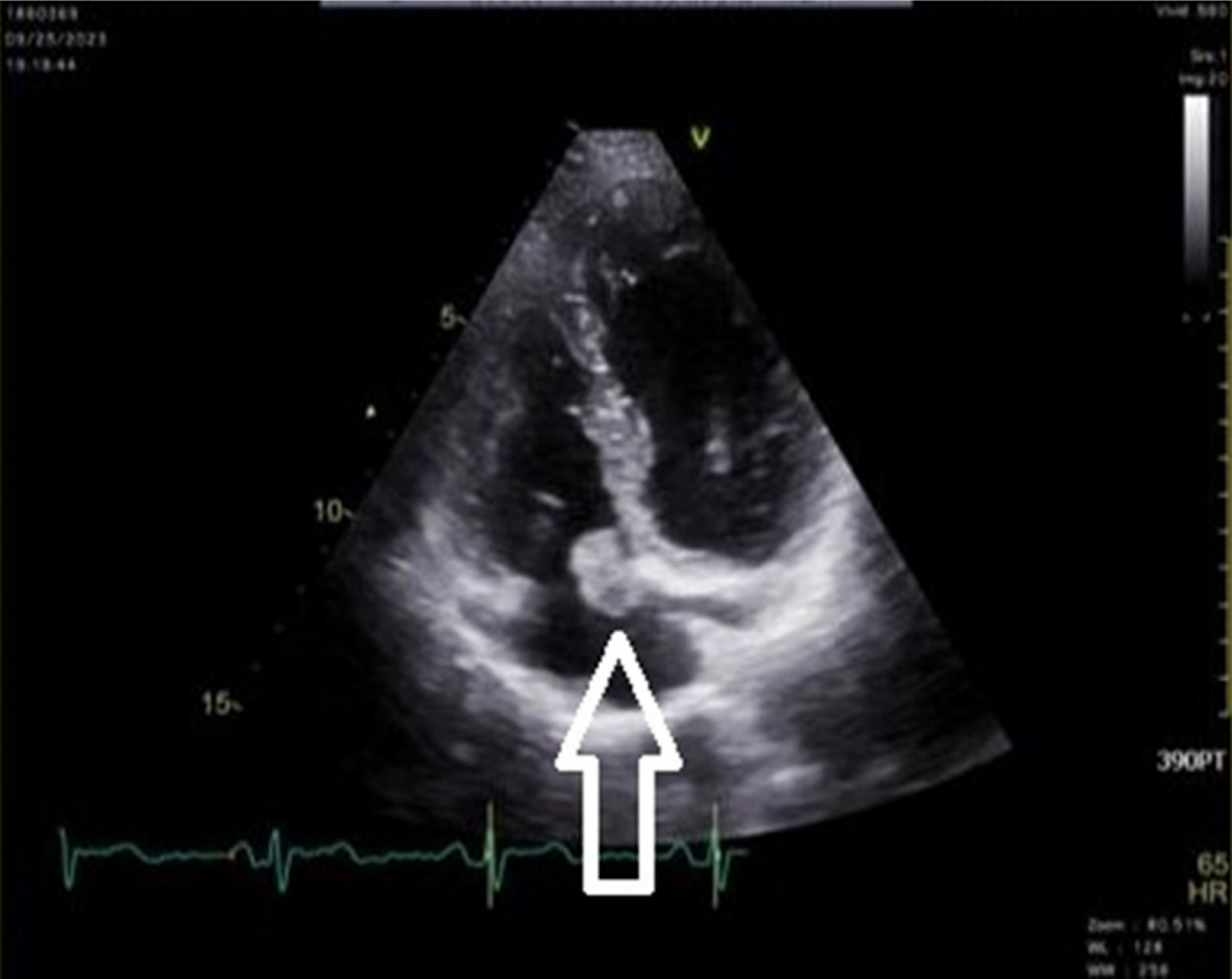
TTE of a 44‐year‐old male with pleuritic chest pain before surgery (4‐chambers view). Large oval‐shaped mobile, gelatinous, and homogenous echodensity (2.0 cm× 1.6 cm) is seen in RA attached to the posterior part of TV annulus just adjacent to CS orifice, attachment to posterior leaflet could not be evaluated in TTE, mostly in favor of myxoma (white arrow). Notably, there are no significant valvular abnormalities or indications of pericardial effusion. Mild AI, MR, PI, and mild to moderate TR are seen, along with LVEF = 55%. AI, aortic insufficiency; CS, coronary sinus; LVEF, left ventricular ejection fraction; MR, mitral regurgitation; PI, pulmonary insufficiency; RA, right atrium; TR, tricuspid regurgitation; TTE, transthoracic echocardiography; TV, tricuspid valve.
